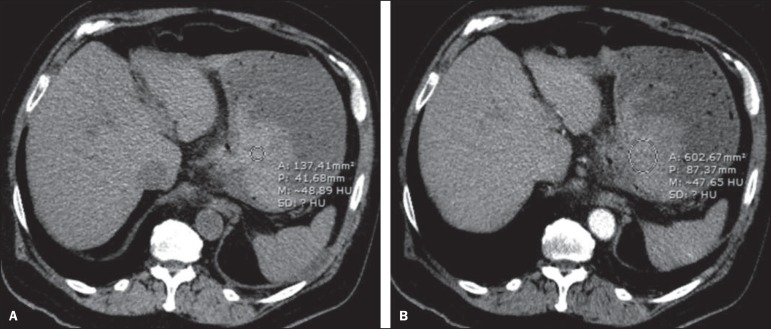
Abdominal CT angiography. Non-contrast-enhanced phase (A)
